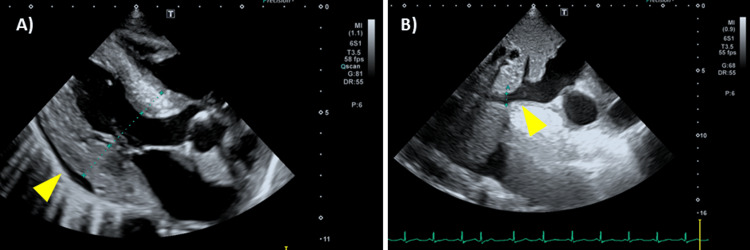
Transthoracic echocardiography on admission. (A) Three-chamber view showing a small pericardial effusion (arrow) and concentric left ventricular wall thickening (IVSd 13.5 mm, PWd 17.5 mm). Left ventricular wall motion is preserved, with an estimated ejection fraction of 60%. No significant valvular disease is observed (mild MR, AR, and TR regurgitation). (B) The inferior vena cava (IVC) diameter is 9 mm, with good respiratory variation (arrow). MR, mitral regurgitation; AR, aortic regurgitation; TR, tricuspid regurgitation; IVSd, interventricular septum diameter; PWd, posterior wall diameter
